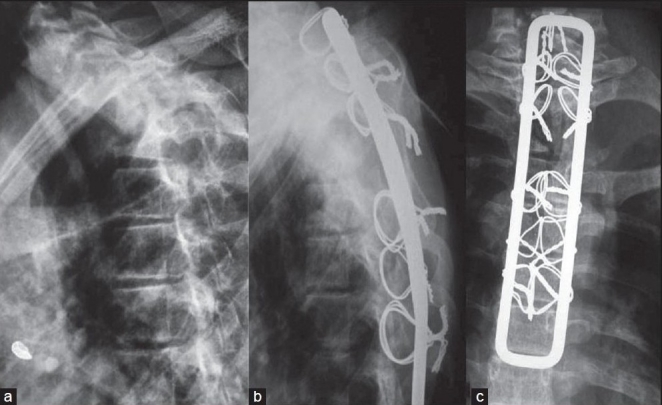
The preoperative lateral X-ray (a) of the same patient shows preoperative kyphosis of 80°. The anterior decompression with kyphus correction and Hartshill fixation was done with extra pleural anterolateral approach. Follow-up lateral X-ray (b) shows corrected kyphosis to 40° and AP X-ray (c) shows resection of second, third and fourth ribs and cortico cancellous grafting in place with Hartshill instrumentation *in situ*
